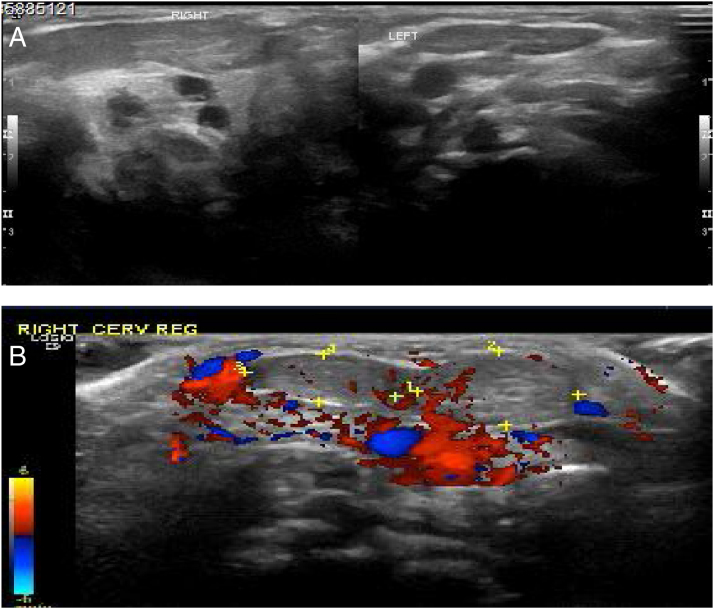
The ultrasound finding on figure A and B demonstrate bulky right sternocleidomastoid muscle as compared to the left side.
